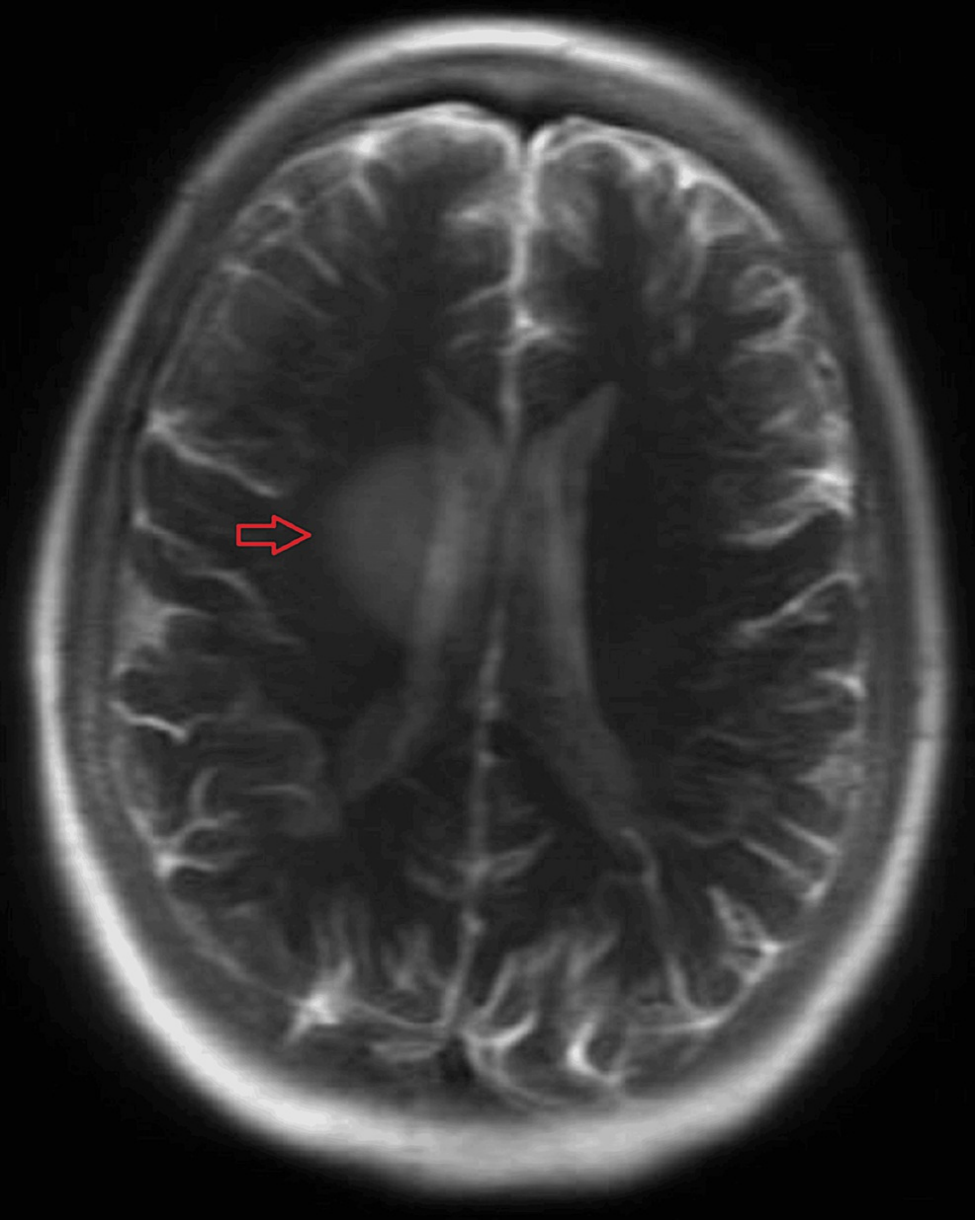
A 2.3 cm rim-enhancing right lateral ventricle periventricular lesion with moderate perilesional edema seen on MRI of the brain Magnetic resonance imaging (MRI)
